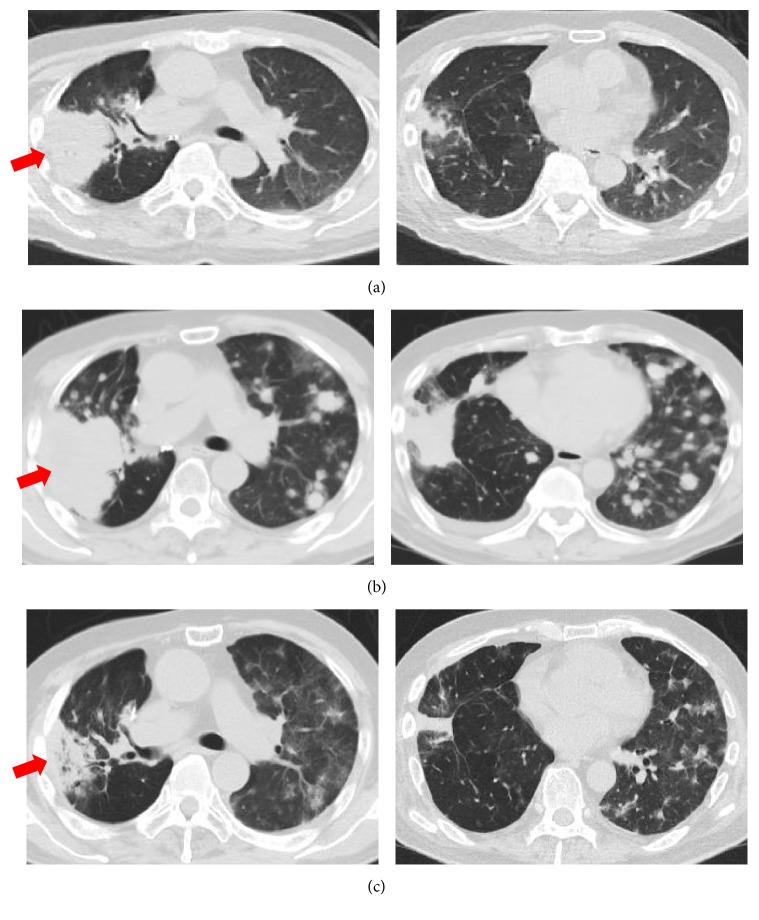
CT scan of the chest (a) before nivolumab treatment, (b) after 3 cycles of nivolumab treatment, and (c) osimertinib day 14.
